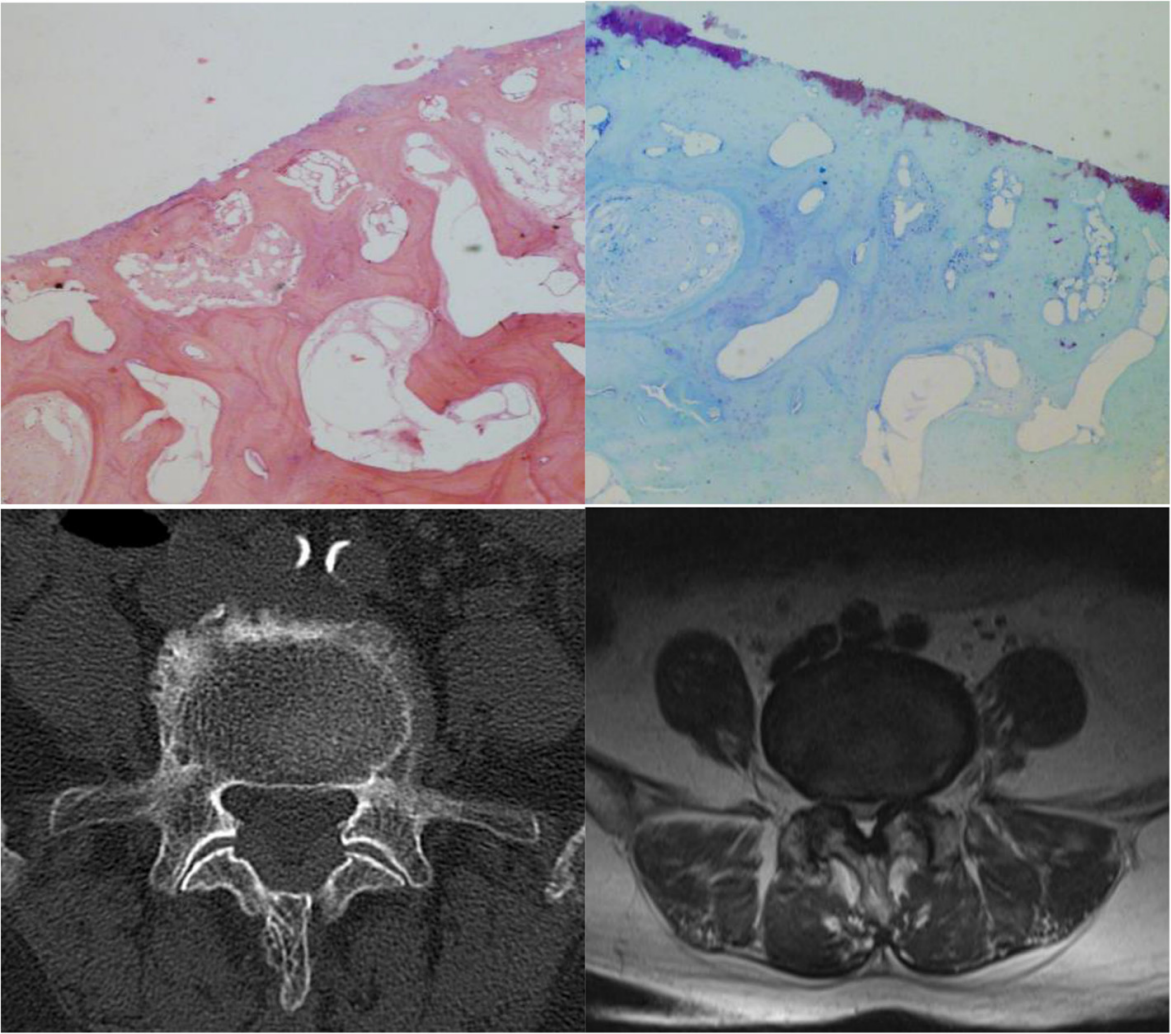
The pathologic grading of the right L4/5 facet joint was 4. While the CT grading was 3, the MR and CT combined MR grading was 4. The CT grading underestimated the degree of facet joints degeneration
